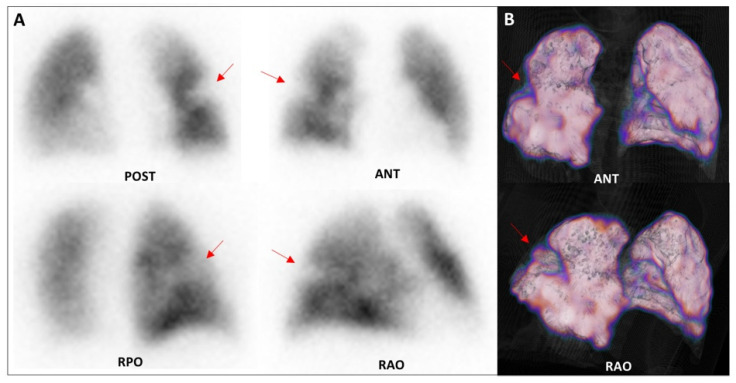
Lung perfusion scintigraphy performed for the suspicion of APE in a 79-year-old patient with dyspnoea, left chest pain, increased d-dimer values (842 ug/L, n.v. < 500), and chronic renal failure. Planar acquisitions (A) and tomographic 3D reconstruction (B) demonstrated a single wedge-shaped subsegmental perfusion defect in the posterior segment of the right upper lobe, thus confirming the suspicion of APE. Consequently, anticoagulant therapy was planned, and after one day of critical parameter monitoring, the patient was discharged early with adequate home therapy. POST: posterior; ANT: anterior; RPO: right posterior oblique; RAO: right anterior oblique.
